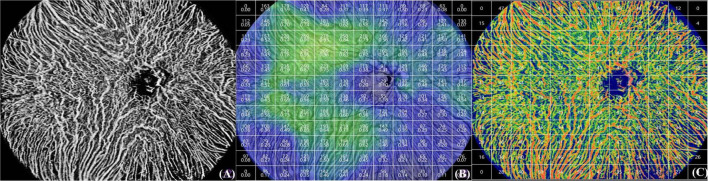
A classic case. (A) Choroidal blood flow structure of 1 patient with high myopia under 24 mm × 20 mm scan; (B) CT; (C) CVI.
